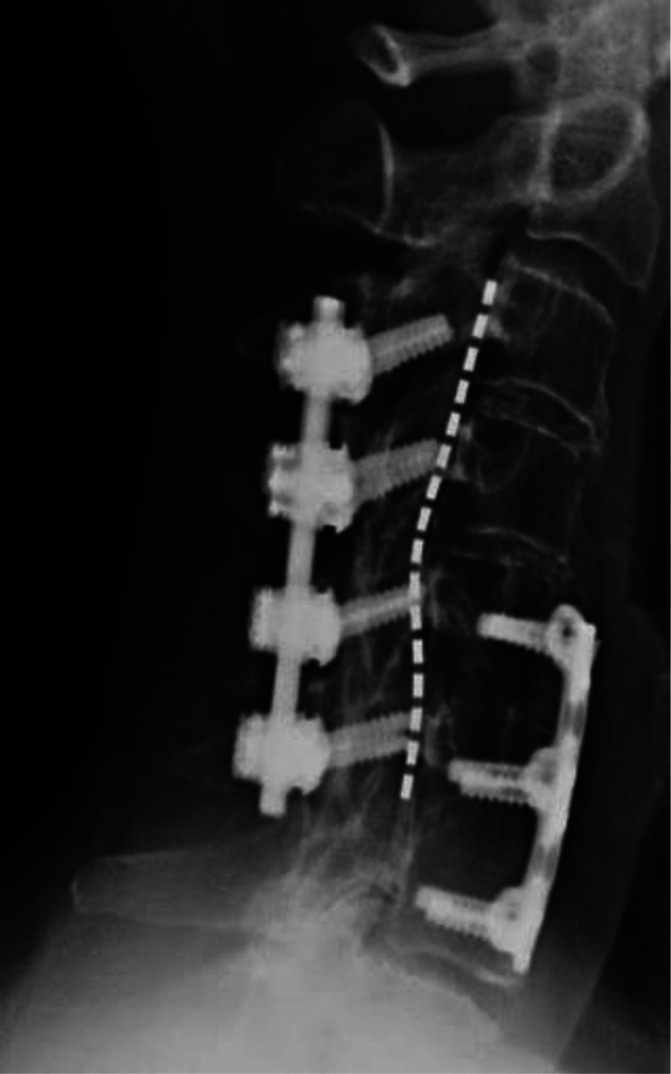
Lateral view x-ray of patient's cervical spine obtained through fluoroscopy. Spinal cord stimulator lead shown to be placed at the bottom of C1 vertebral body with anterior and posterior hardware present.
